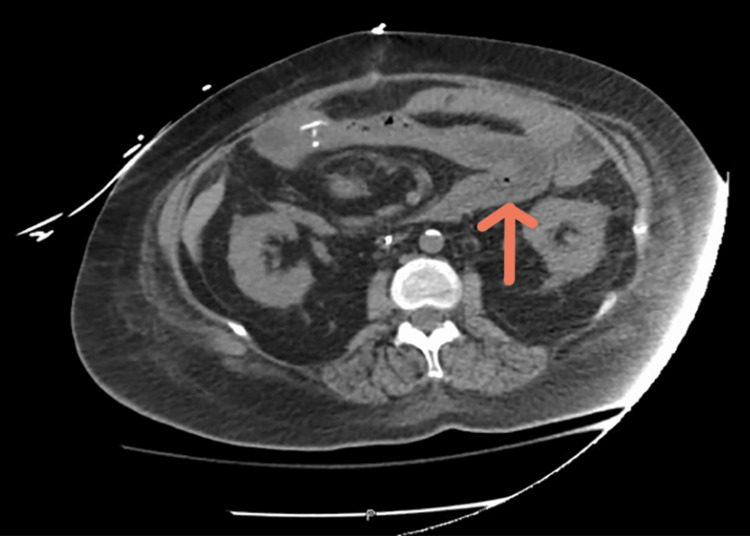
CT scan of the abdomen showing widespread ischemic bowel
